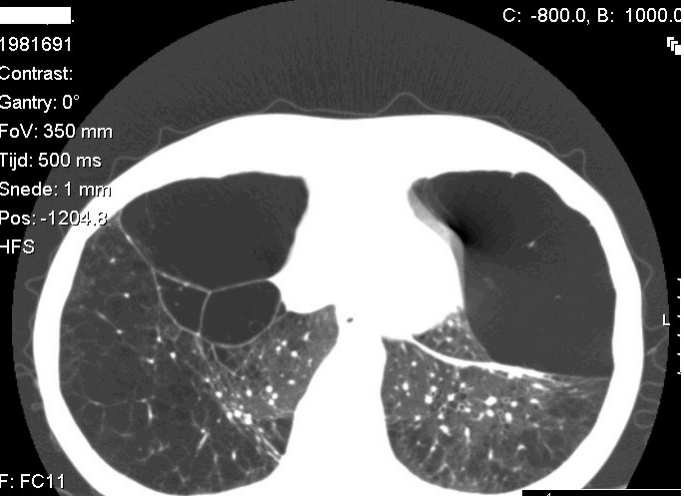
Computed tomography of the lung with thin slices (1 mm) showing emphysema and bullae in the lower lung lobes of a subject with type ZZ alpha-1-antitrypsin deficiency. There is also increased lung density in areas with compression of lung tissue by the bullae.
